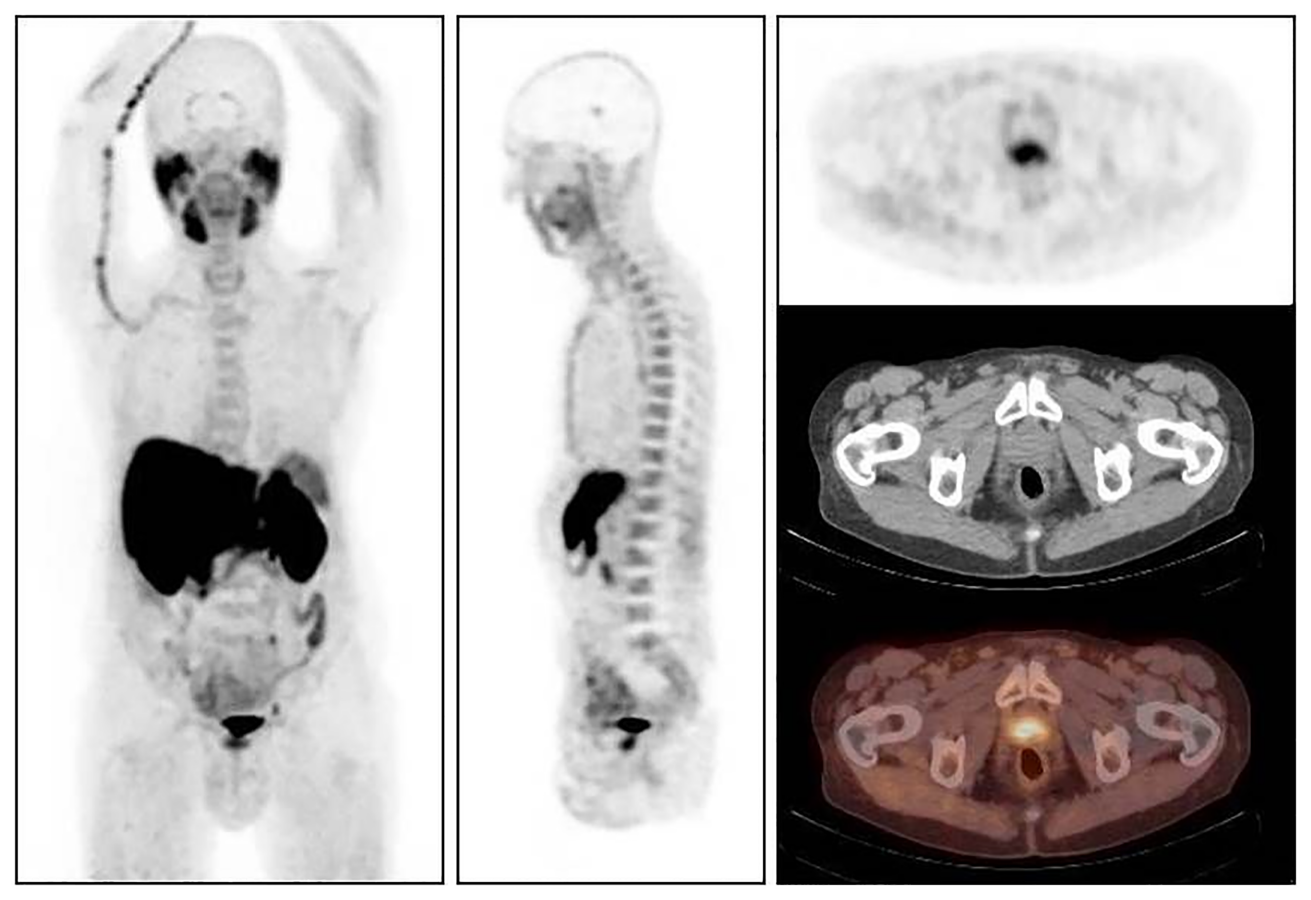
76y old patient with prostate cancer Gleason score 7, treated 5 years ago by high intensity focused ultrasound (HIFU). Serum PSA level at the time of FCH PET/CT 9.1ng/mL. FCH PET/CT revealed isolated FCH uptake by two lobes of prostate but no foci evocative of regional of distant prostate cancer recurrence. Scheduled palliative medical treatment was replaced by radiation therapy with curative intent. Adequacy of this therapeutic decision was confirmed by clinical follow-up, serum PSA level 11 months after FCH PET/CT = 0.8 ng/mL.
